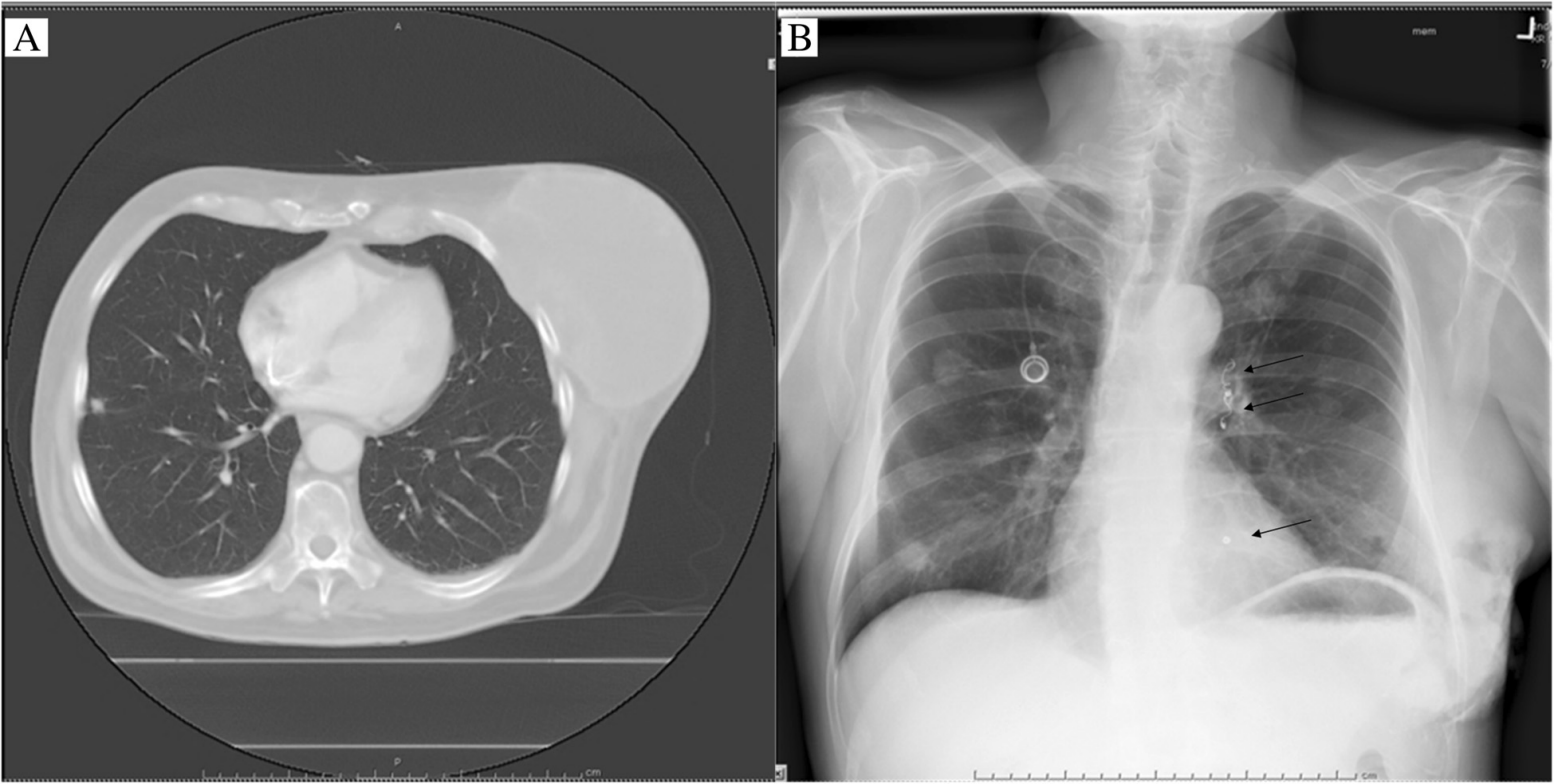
Pretreatment chest CT scan (A) showing left chest wall sarcoma and preoperative chest x-ray (B) with evidence of tumor and multiple embolization coils (dark arrows).
